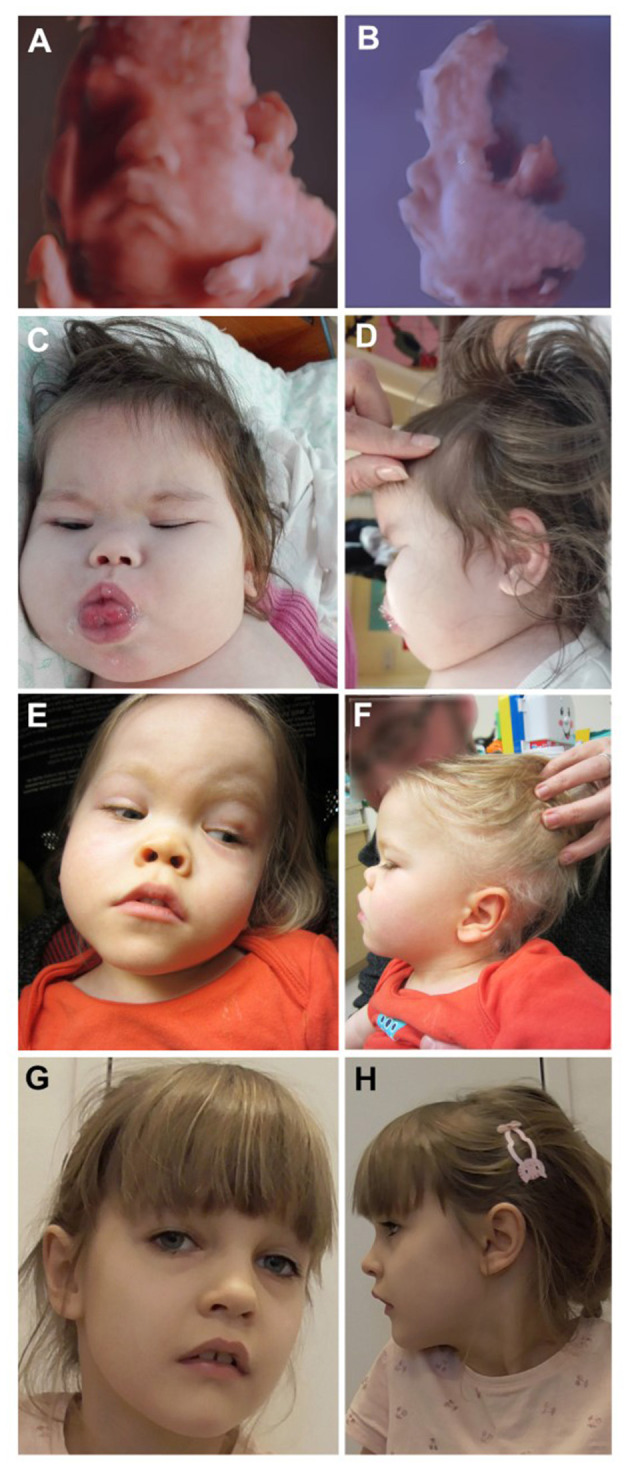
(A) Prenatal 3D ultrasound of patient 2 at 34 weeks 2 days' gestational age, frontal view showing carpe-shaped mouth with tenting of both upper and lower lip. (B) Prenatal 3D ultrasound of patient 2 at 34 weeks 2 days' gestational age, lateral view showing craniofacial features with depressed nasal bridge, upturned nasal tip and prominent upper lip. (C) Facial features of patient 4 at age 10 months, frontal view showing depressed midface with full cheeks and prominent philtral ridges. (D) Facial features of patient 4 at age 10 months, lateral view showing deep-set eyes and low-set ears. (E) Facial features of patient 1 at age 3 years, frontal view showing high forehead, depressed midface, full cheeks, and tented mouth. (F) Facial features of patient 1 at age 3 years, lateral view showing deep-set eyes, upturned nasal tip, and low-set and posteriorly rotated ears. (G) Facial features of patient 5 at age 7 years, frontal view showing minimal dysmorphic features with tented upper lip and widely spaced teeth. (H) Facial features of patient 5 at age 7 years, lateral view showing mild posterior rotated and low-set ear and prominent, short, philtrum.
